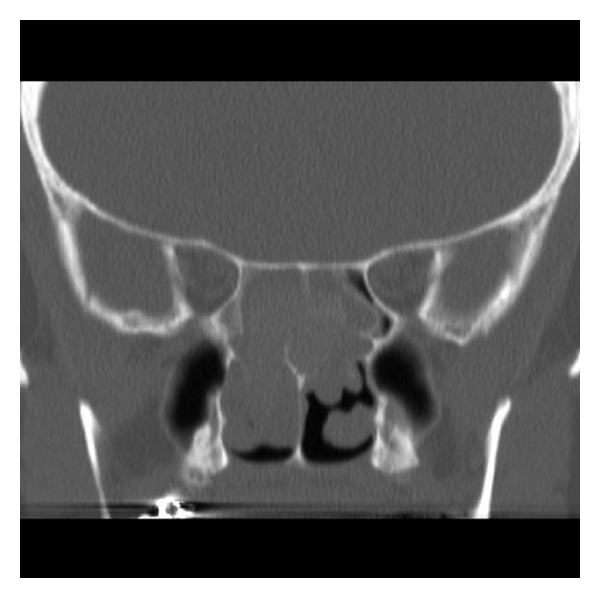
Coronal CT image showing bilateral opacification and polyposis in both sphenoethmoidal recesses.
